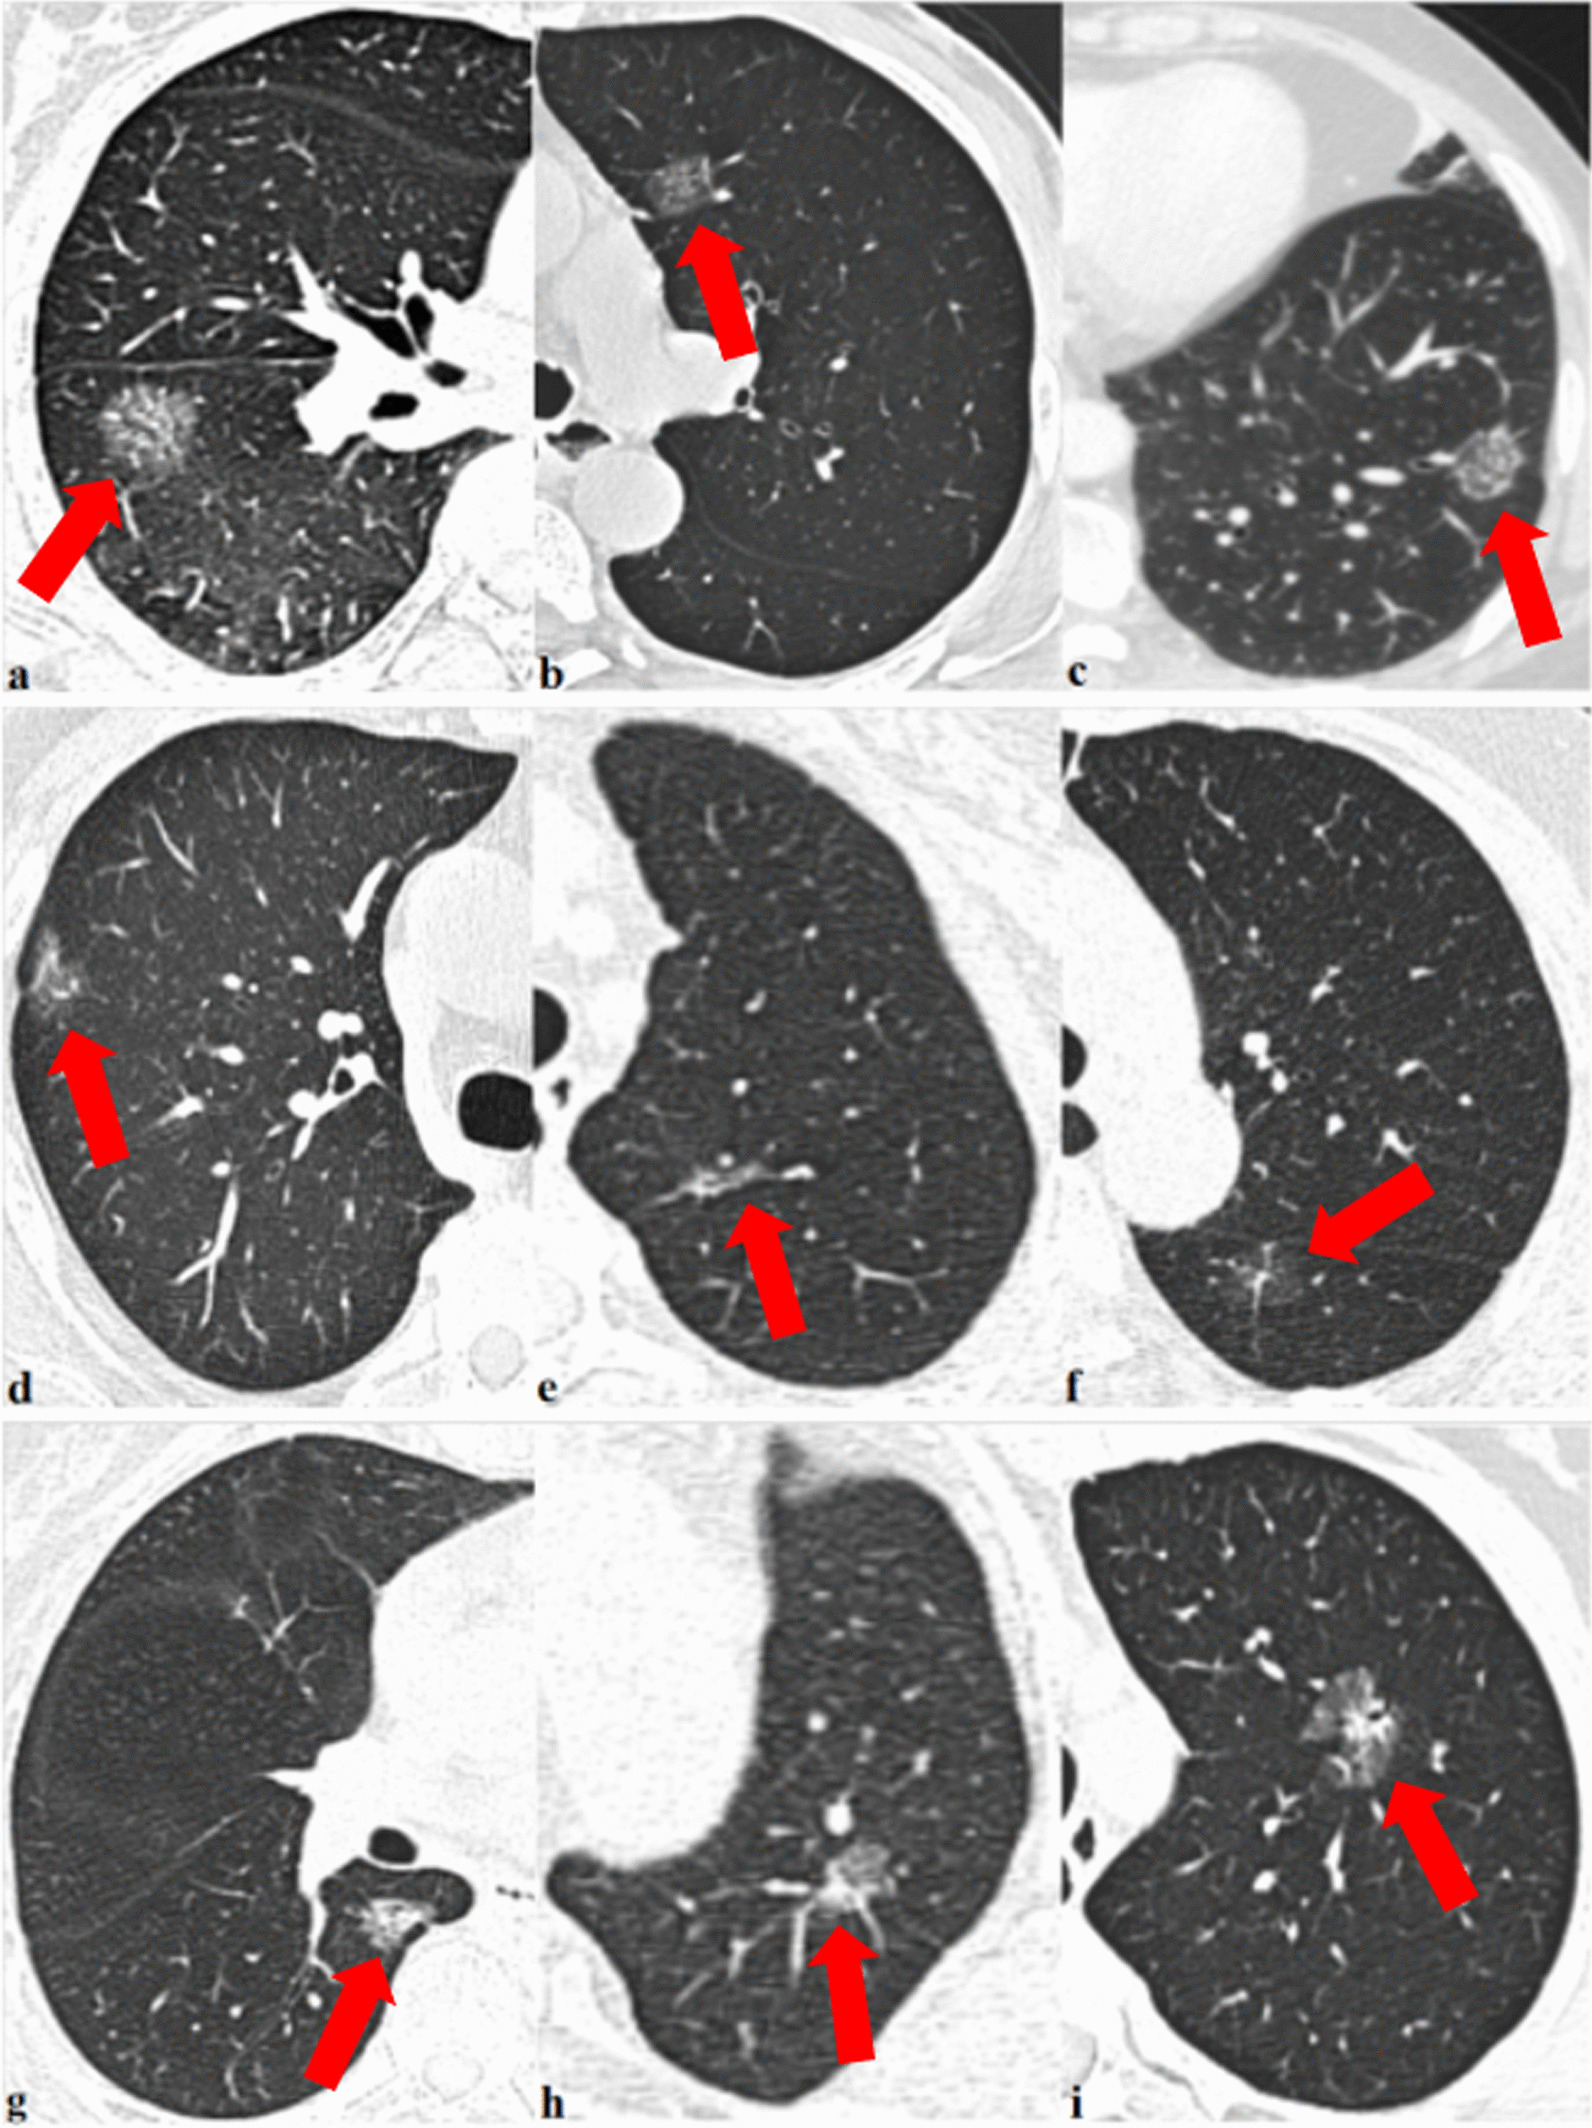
Axial computed tomography images show examples of solid component morphology, including the following: punctate consolidations smaller than 5 mm in part-solid nodules (a–c); stripe consolidations larger than 5 mm in part-solid nodules (d–f); irregular consolidations larger than 5 mm in part-solid nodules (g–i)
